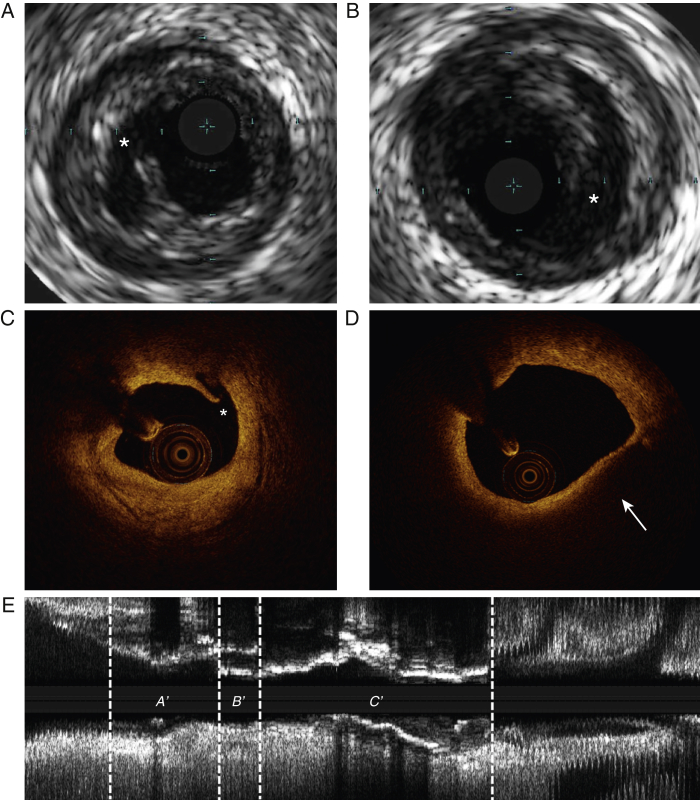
Identification of Plaque Rupture Using Gray-Scale Intravascular Ultrasound and Optical Coherence Tomography (A and B) Gray-scale IVUS images of spontaneous plaque rupture (*). (C) OCT image showing evidence of rupture (*).(D) Thin-cap fibroatheroma (arrow) as identified by OCT with no evidence of rupture or erosion. (E) Longitudinal IVUS reconstruction of a coronary artery with evidence of plaque rupture; proximal (*A′*); rupture site (*B′*); and distal (*C′*) segments. IVUS = intravascular ultrasound; other abbreviation as in Figure 1.
